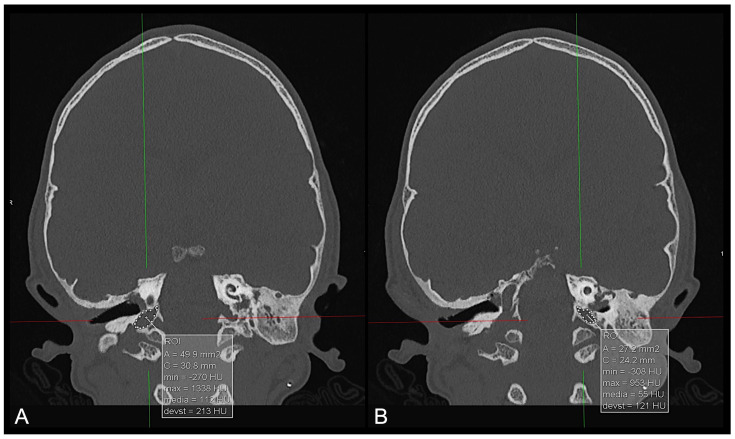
CT multiplanar coronal reconstruction of the jugular foramina in a patient diagnosed with brachycephaly showing a larger right jugular foramen ((A)—approximately 50 mm^2^) than on the left side ((B)—approximately 27 mm^2^) by means of area calculations obtained by placing manual regions of interest (ROIs—white dotted lines).
